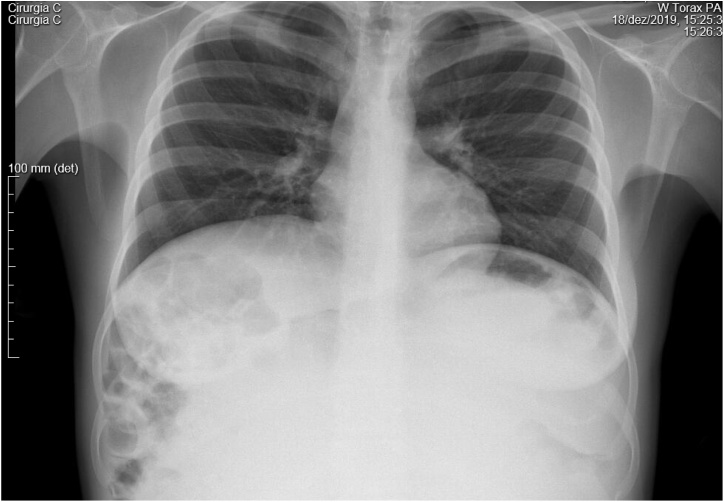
Thoracic X-ray showing symmetrical elevation of both diaphragmatic domes and presence of collapsed small bowel in the right upper abdominal quadrant.
